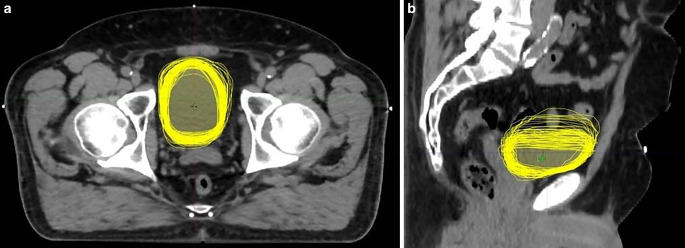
All bladder contours taken during the whole treatment course of a patient, shown on the planning CT. With rigid registration to the bony structures. a Axial view, b sagittal view
